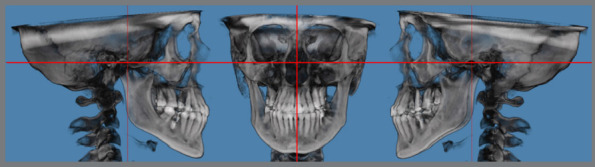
Orientation of the three-dimensional model from CBCT. The horizontal red line represents the Frankfurt plane, and the vertical red line represents the Nasio-Palatal line.
